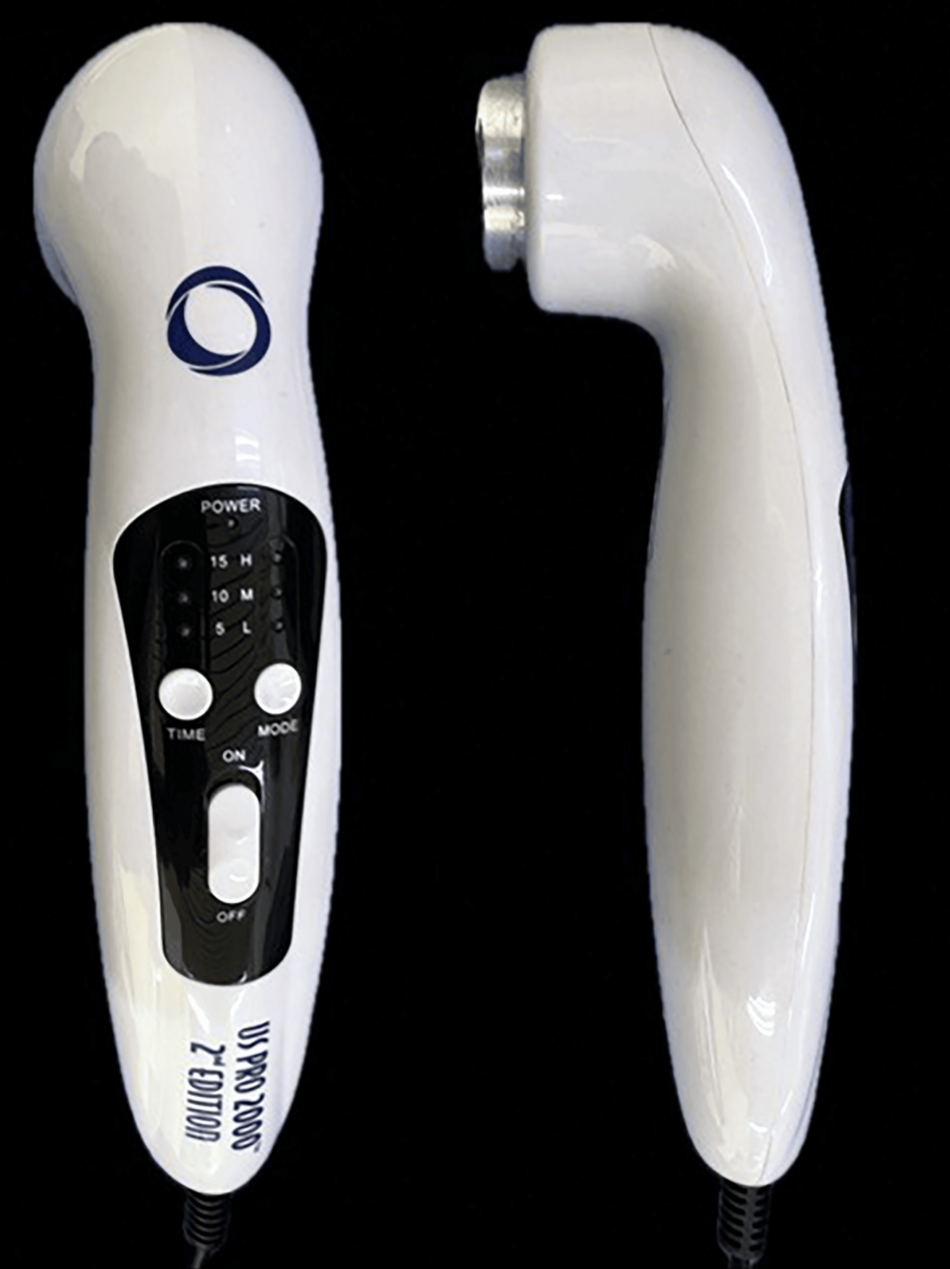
The low-intensity pulsed ultrasound (LIPUS) device that was used in the current study.
